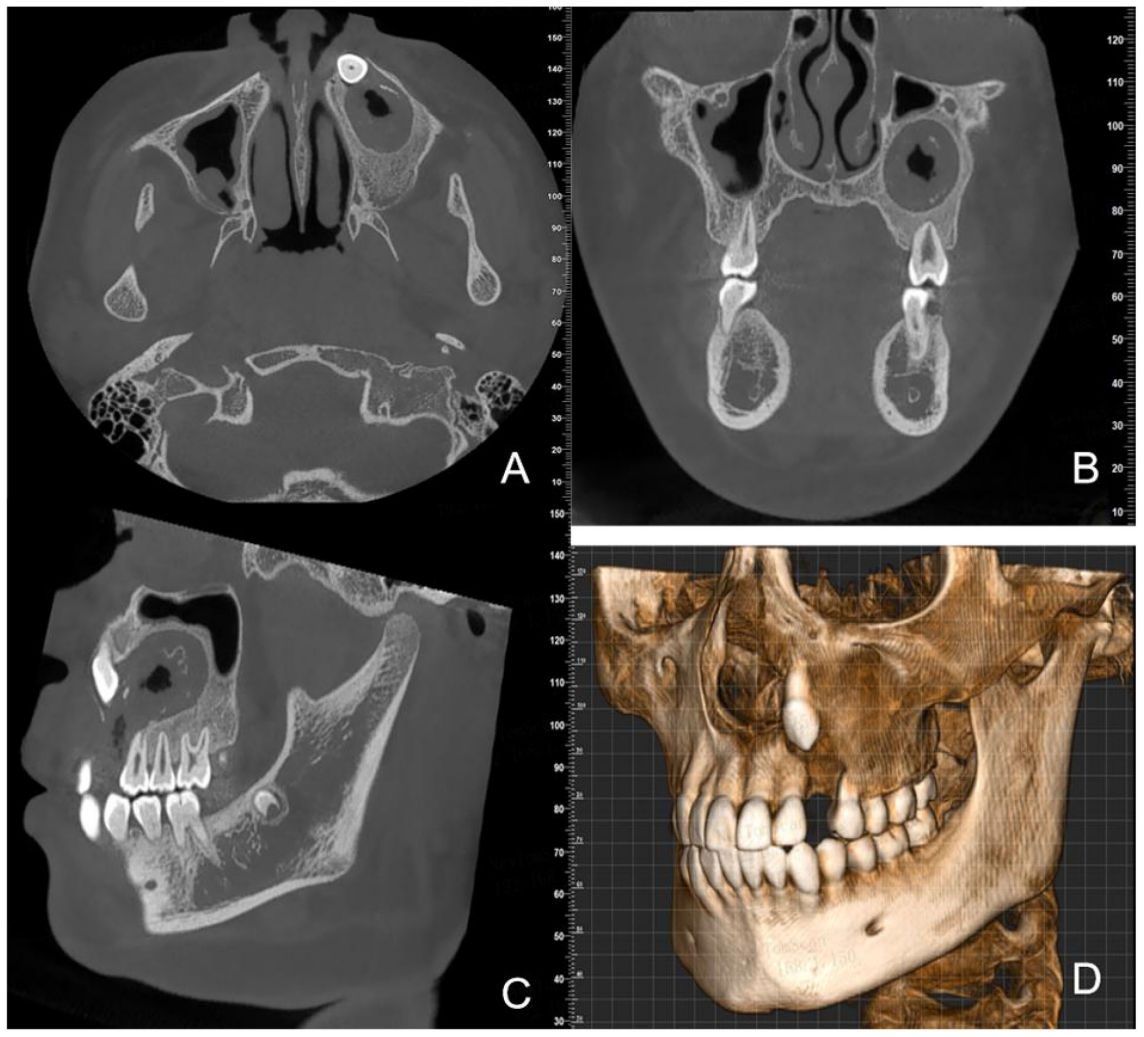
CBCT images one year after decompression surgery (A) (axial view) (B) (coronal view) (C) (sagittal view) (D) (3D reconstruction) show continued lesion reduction and ongoing bone repair.
